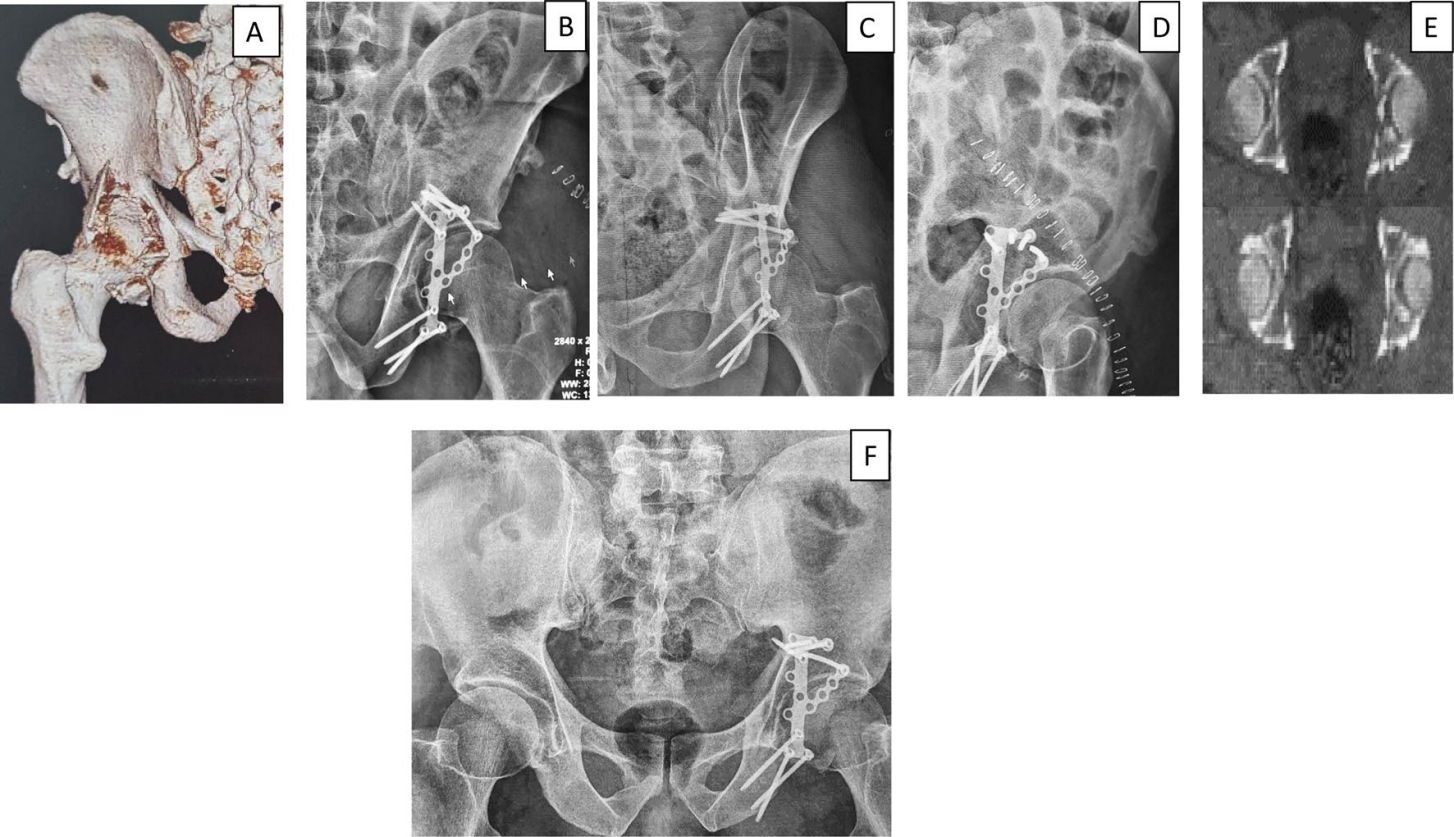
A 3D CT scan of 46 year-old male patient showing comminuted PW fracture of the left hip. B, C, D Postoperative radiograph (AP and Judet views) after calcaneal plate fixation showing anatomically reduced fracture. E CT (axial cuts) after fixation showing anatomical reduction. F 1 year radiographs show stable fixation with no reduction loss or fixation failure
